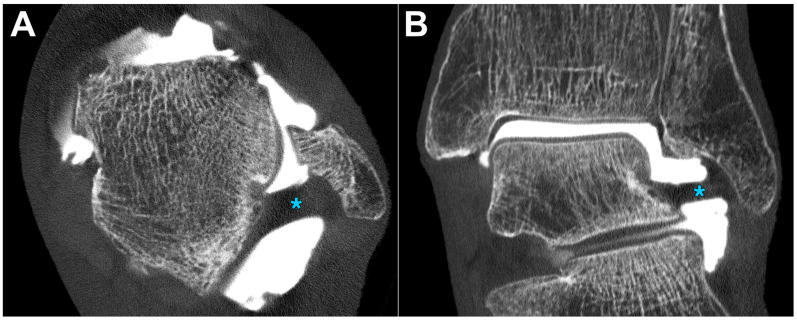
Depiction of an intact posterior tibiofibular ligament (blue asterisk) in axial (A) and coronal orientation (B). Photon-counting CT arthrography was performed with a CTDI_vol_ of 10 mGy. The acquired dataset was reconstructed with the ultra-sharp Br98 kernel, which possesses the highest spatial frequency of all convolution kernels available for ultra-high-resolution imaging on a photon-counting detector.
